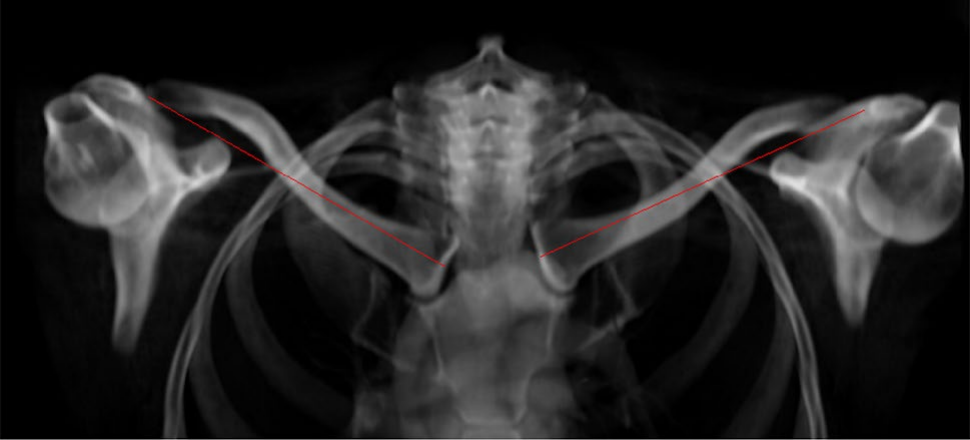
Example of measurements on 3D reconstruction of a CT scan showing both clavicles
